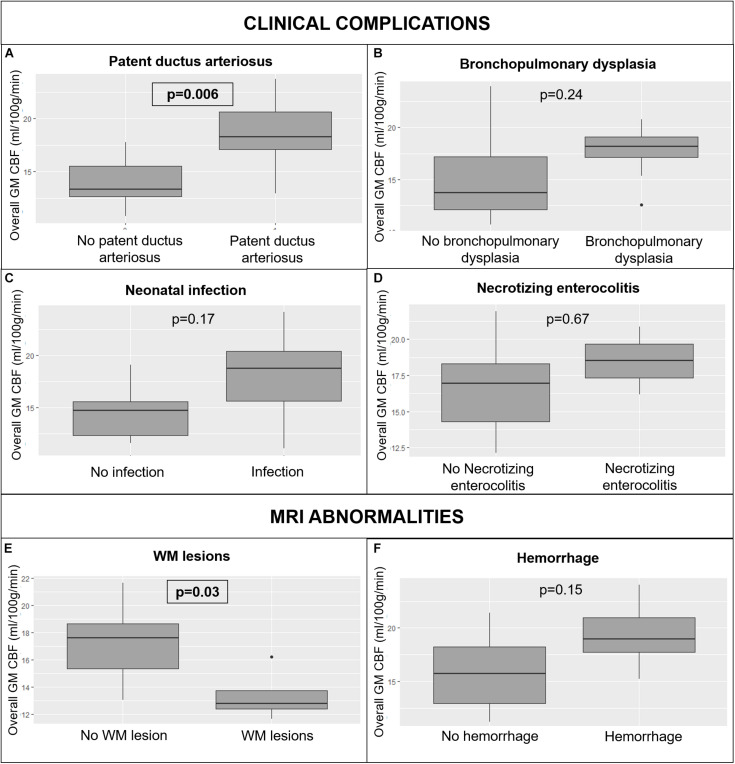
Variations in whole brain GM CBF in preterm infants according to clinical complications: patent ductus arteriosus (A), bronchopulmonary dysplasia (B), neonatal infection (C), and necrotizing enterocolitis (D); and MRI abnormalities: WM lesions (E) and hemorrhage (F). CBF: cerebral blood flow, MRI: magnetic resonance imaging, WM: white matter. The significant *p*-values are in bold characters within a square frame.
